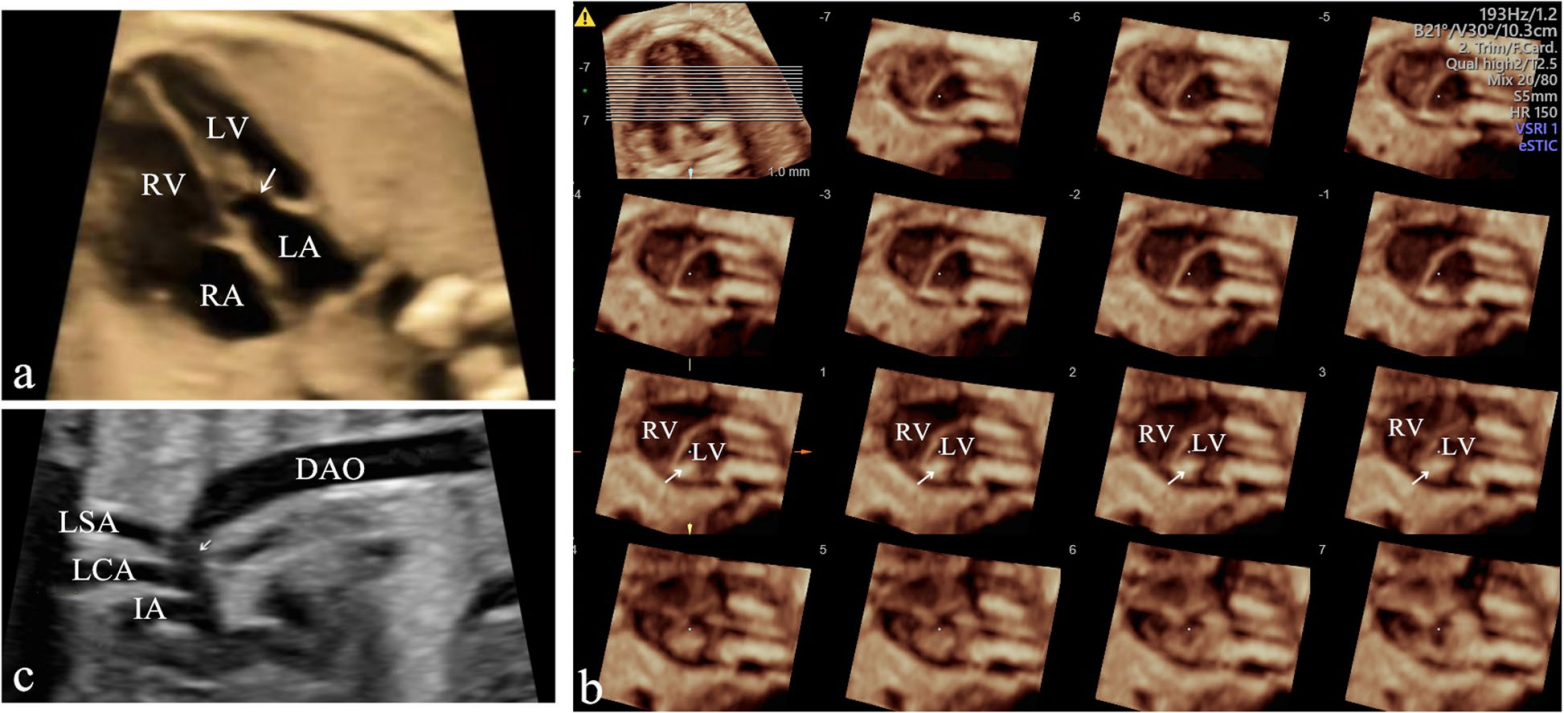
Fetal echocardiographic images. a The four-chamber view showing the shape and limited opening of the mitral valve, which has thickened chordae that converge into a single papillary muscle (PM) in diastole, similar to “Ω” shape (arrow). b Four-dimensional tomographic ultrasound images with short-axis views of the left ventricle at different levels that detected the posteromedial PM (arrow) without anterolateral PM. c Long-axis view of the aortic arch showing marked narrowing of the arch and isthmus (arrow). DAO, descending aorta; IA, innominate artery; LA, left atrium; LCA, left common carotid artery; LSA, left subclavian artery; LV, left ventricle; RA, right atrium; RV, right ventricle
